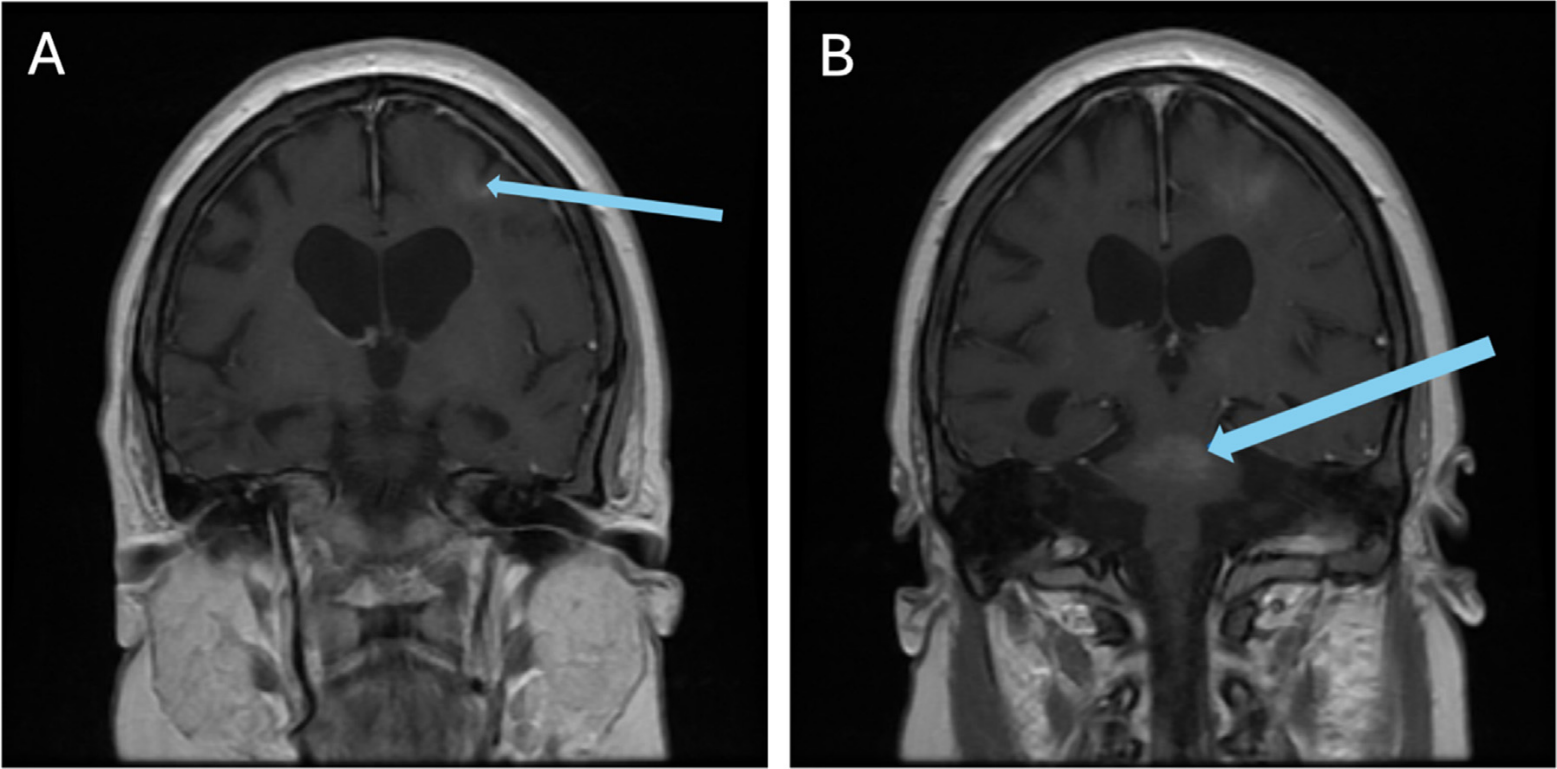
Pre-transplant magnetic resonance images demonstrating low grade enhancement in the precentral gyrus of the left frontal lobe extending in deep aspects of cortex asymmetrically (A) and stippled enhancement in dorsal aspect of mid pons (B) on post-gadolinium images.
